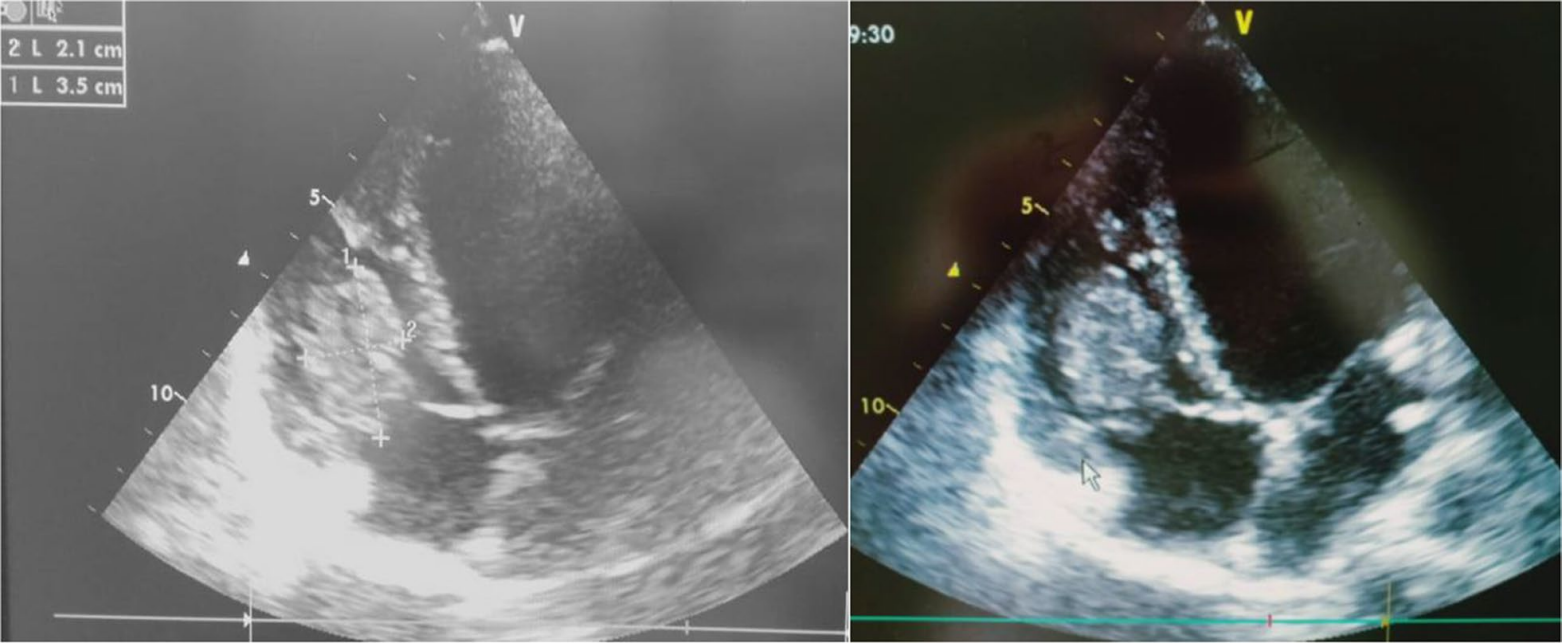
Transthoracic echocardiography: 4-chamber view showing a right ventricle mass attached to the anterior cusp of the tricuspid valve
